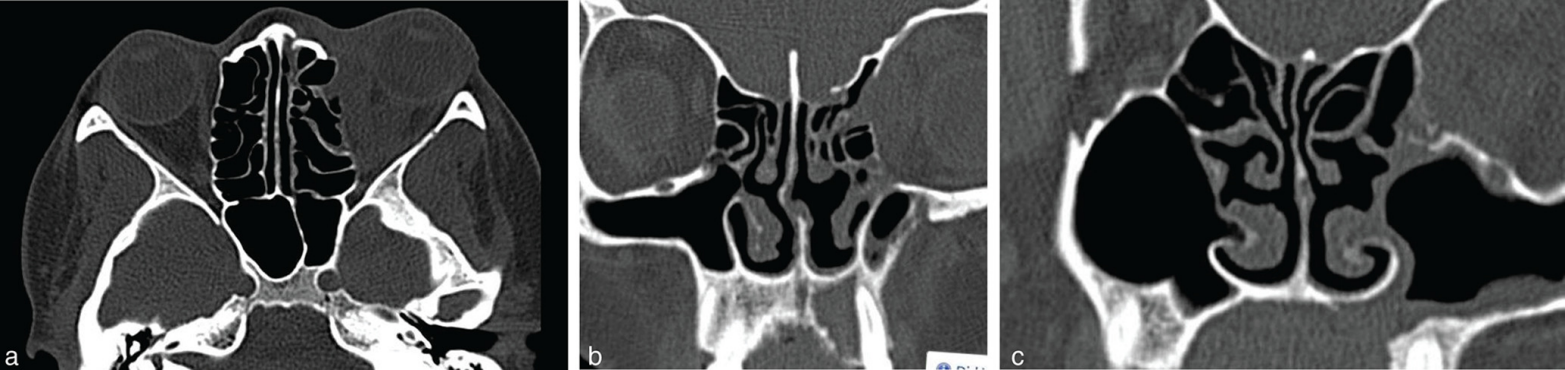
(a–c) Axial and coronal CT images of the paranasal sinuses and orbits demonstrate mild mucosal thickening of the left maxillary (c) and left ethmoid (a, b) sinuses, proptosis of the left globe, retro-orbital fat stranding, soft tissue thickening along the medial wall of the orbit and subtle bony erosion in the left lamina papyracea (a), bony defect in the alveolar margin of the maxilla at the root of the left incisor tooth (b) and an oroantral fistula (c).
